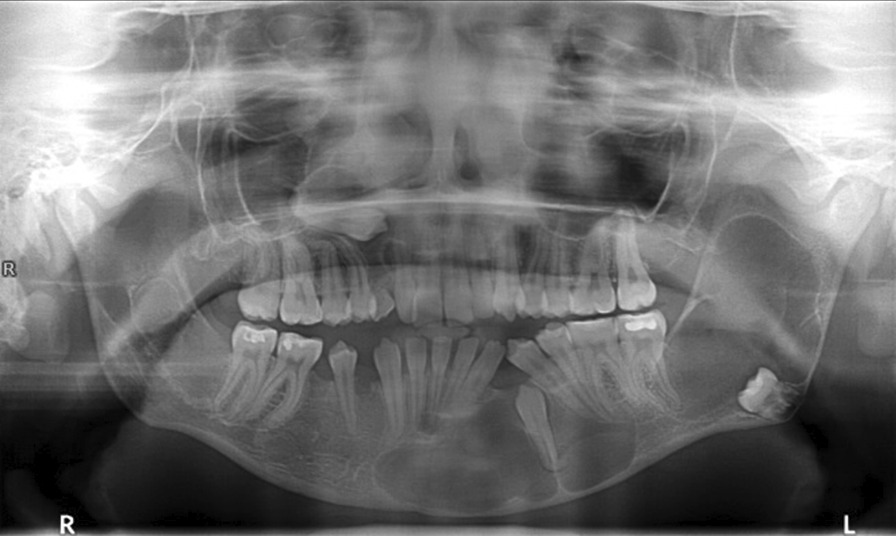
Orthopantomography—4 years after the first surgery (January 2017): enlargement of the lesions in the left mandibular body and ramus; trabeculae in the post-operative cavities
